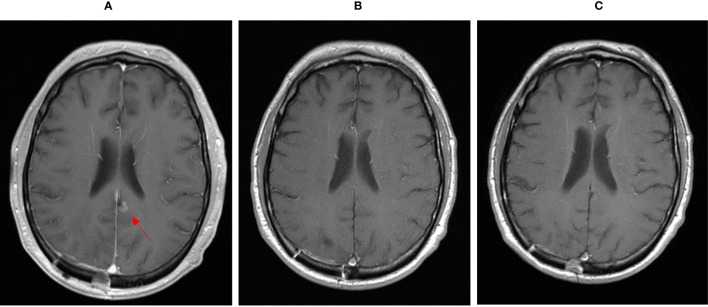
MRI showing cerebral metastasis before (A), after three month (B) and after one year (C) of double check point inhibition with ipilimumab and nivolumab.
